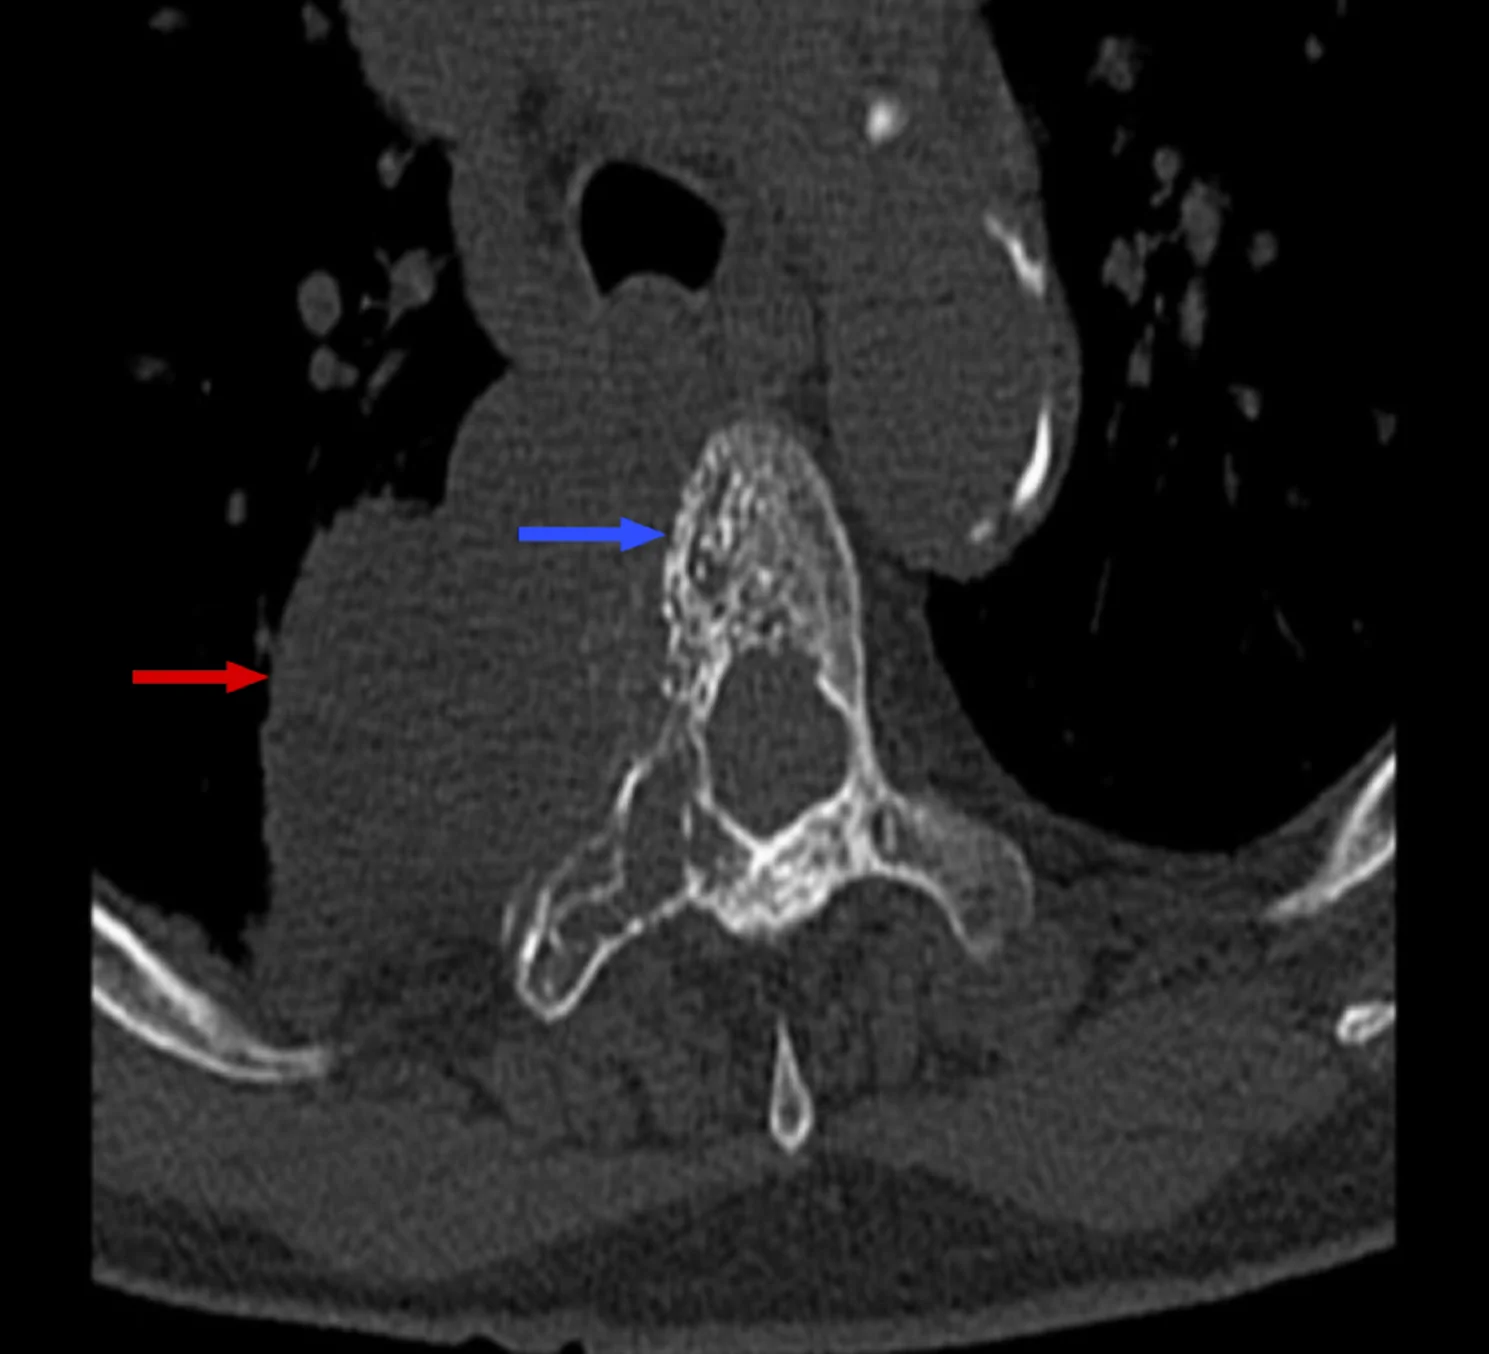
Computed tomography of the thoracic spine demonstrates a heterogeneously enhancing soft tissue lesion (Red arrow) extending from D3 to D6 with right paraspinal, extradural, and neural foraminal extension. The D4 vertebral body shows coarse, heterogeneous trabecular density and bony expansion (Blue arrow)
